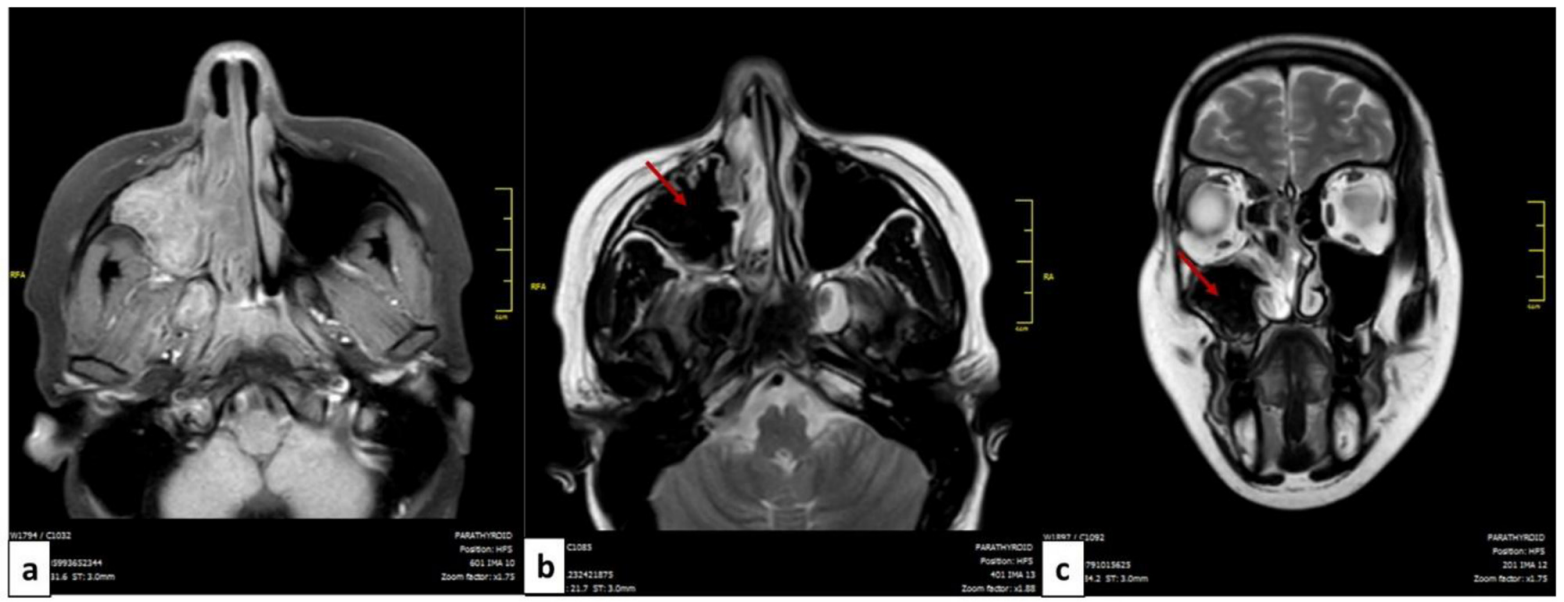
MRI of the facial mass of a patient with allergic fungal rhinosinusitis: axial cut (
